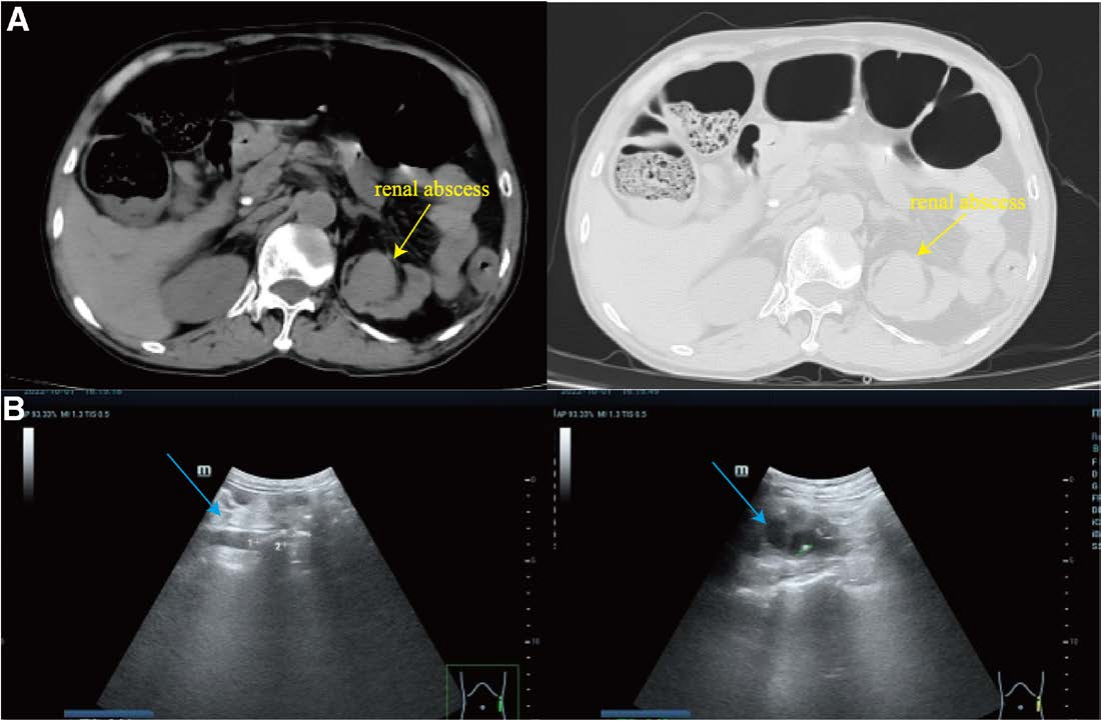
(A) Abdominal CT (the 2nd day after surgery): nodular hyperdense shadow with a diameter of approximately 17 mm × 8 mm × 7 mm was seen in the upper part of the left ureter, above which fluid is accumulated in the left ureter and the left kidney, with a CT value of about 38 HU, quasi-circular low-density foci was seen in the left kidney parenchyma, with a diameter of about 38 mm and a CT value of about 2 HU. (B) Urological ultrasound (4 mo after discharge): the left kidney is still normal in size, the parenchyma is thin, and the internal structure is still clear. CT = computed tomography.
